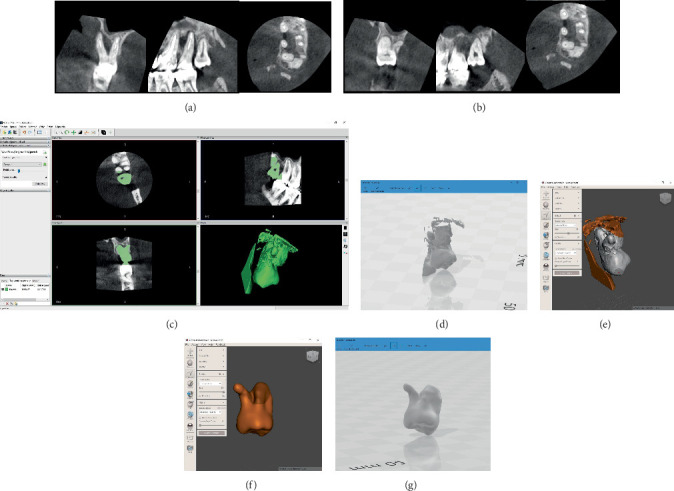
Preoperative planning. The CBCT examination revealed the (a) failed Tooth #15 and (b) donor Tooth #16 with multiple conical fused roots and a buccally placed root measuring 3 mm, angled at 90°. (c–g) The replica was crafted after sectioning the tooth from the CBCT scan and smoothing its surface.
